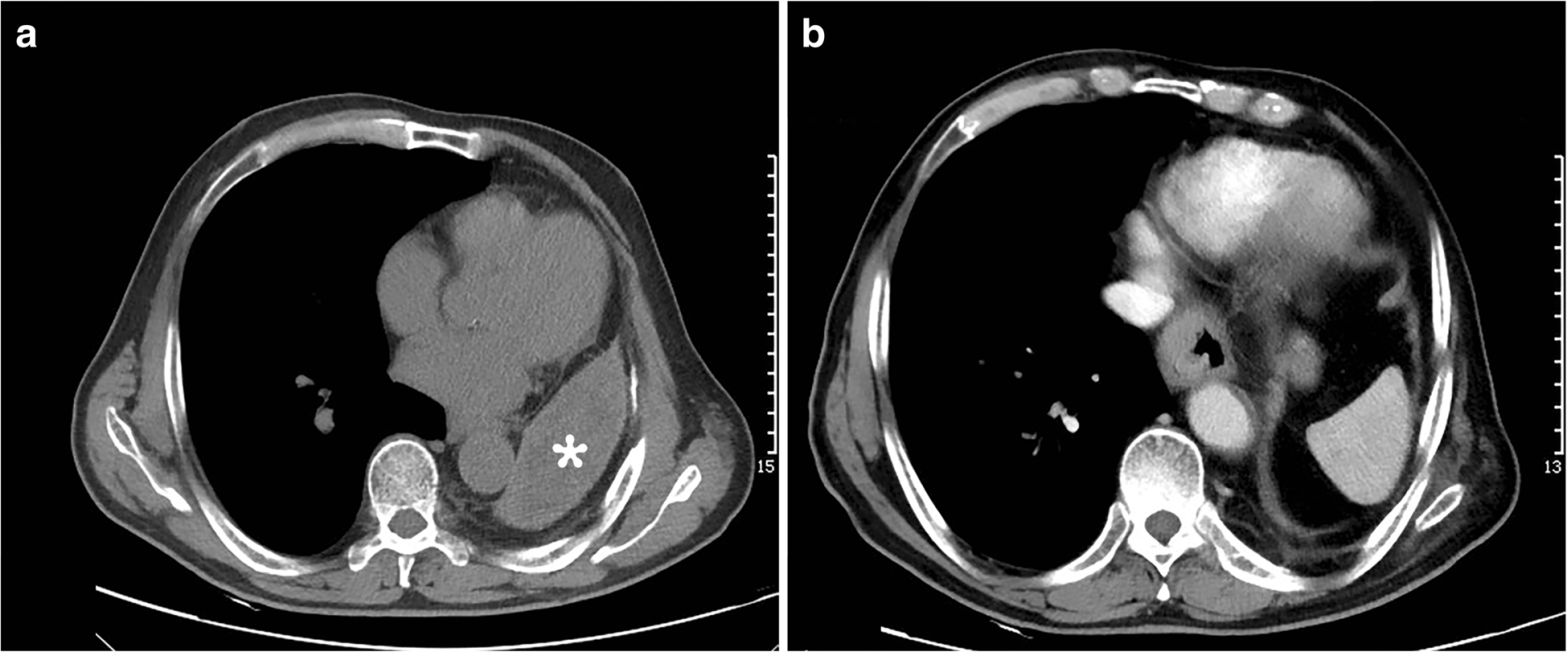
Preoperative computed tomography imaging of the patient showed typical postpneumonectomy changes, characterized by a hyperexpansion of the residual lung, mediastinal shift to the opacified postpneumonectomy space (asterisk) as well as b elevation of the hemidiaphragm superior to the level of the esophageal mass
